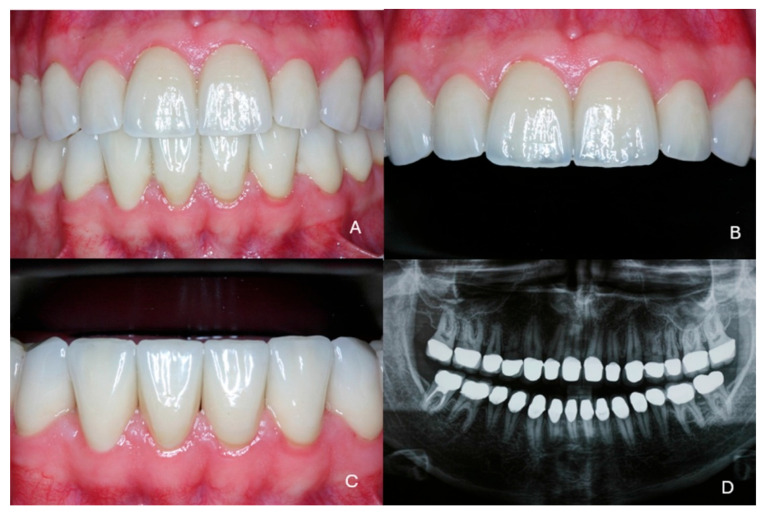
The final clinical situation at the end of the treatment. (A) Frontal view, (B) maxillary anterior teeth, (C) mandibular anterior teeth, (D) radiographic examination.
